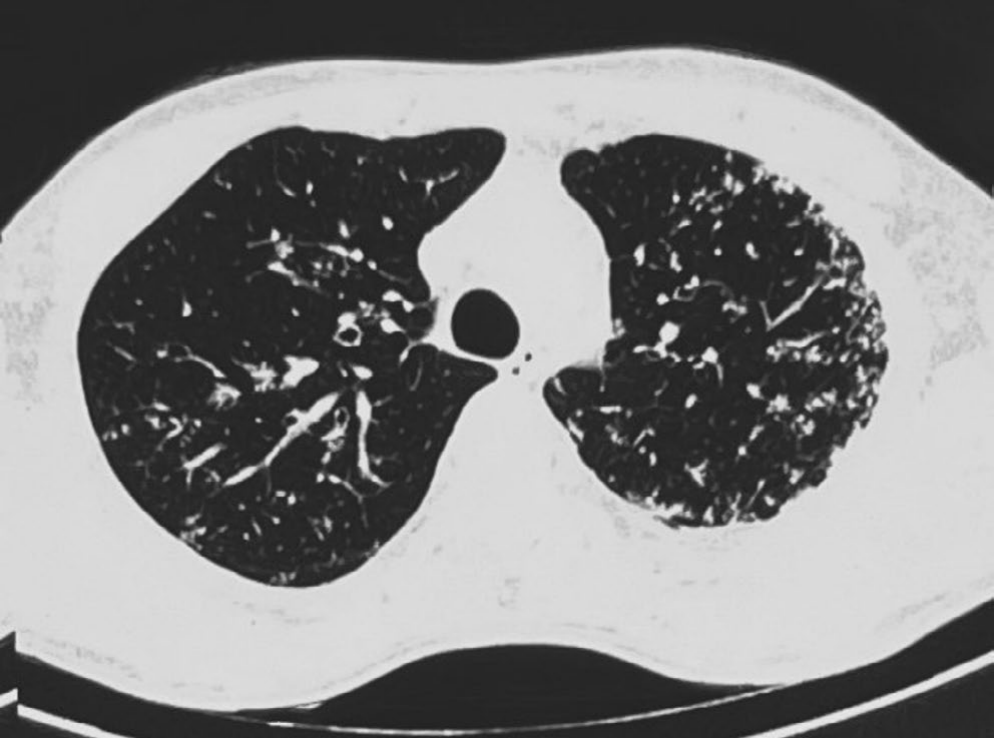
Computed tomography (CT) of the chest showing diffuse tree‐in‐bud opacities in bilateral lung fields associated with minimal pleural effusion.
